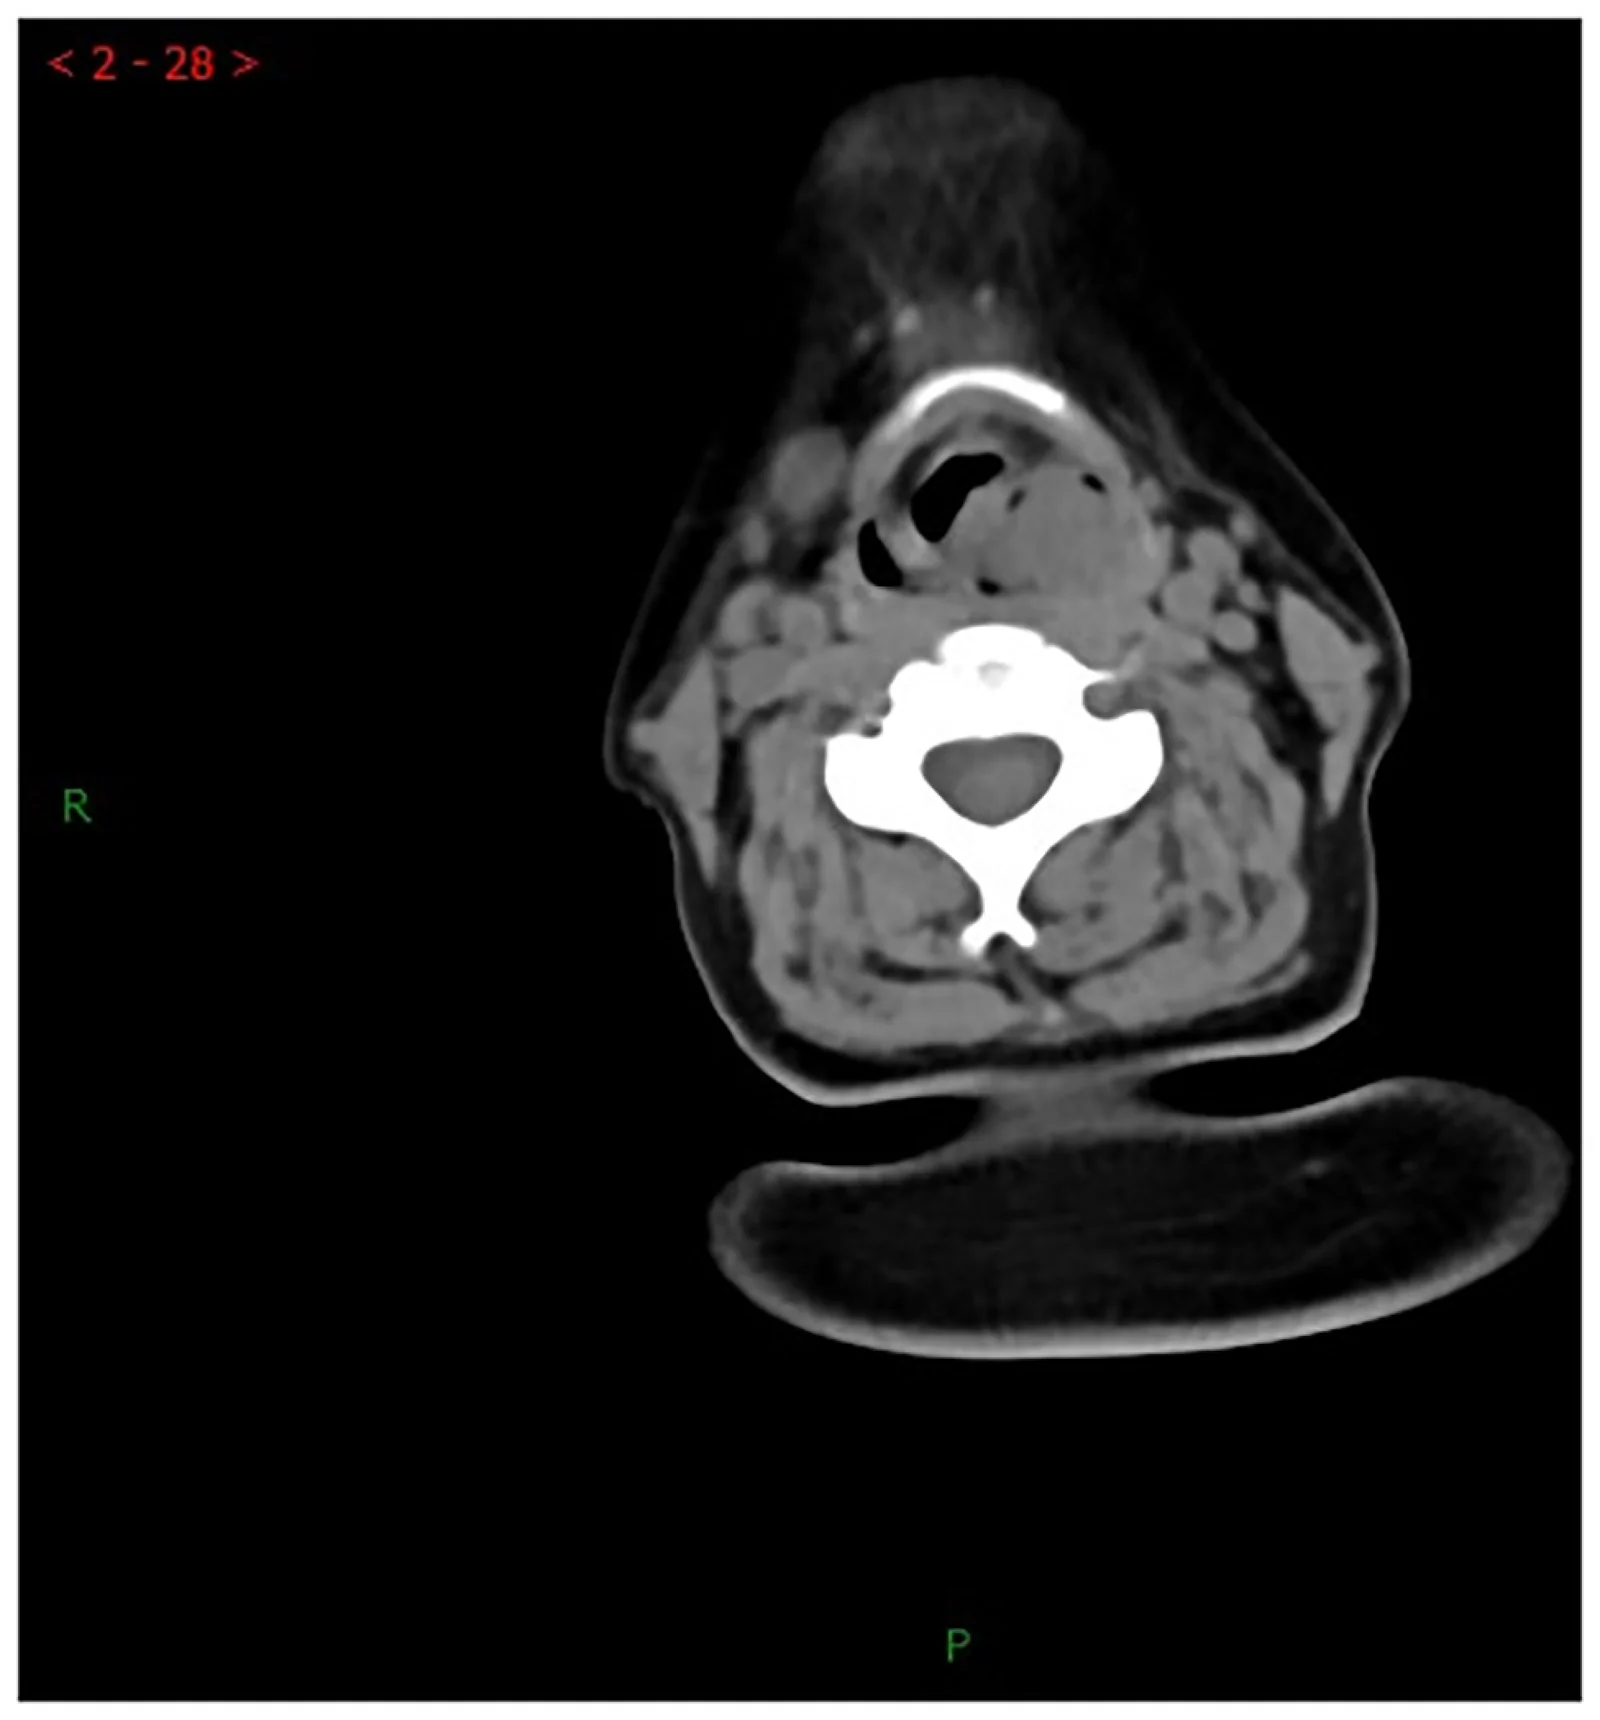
Neck CT findings. An ill-defined, heterogeneously hypodense mass in the left pyriform sinus, measuring 32 mm × 27 mm. CT = computed tomography.
